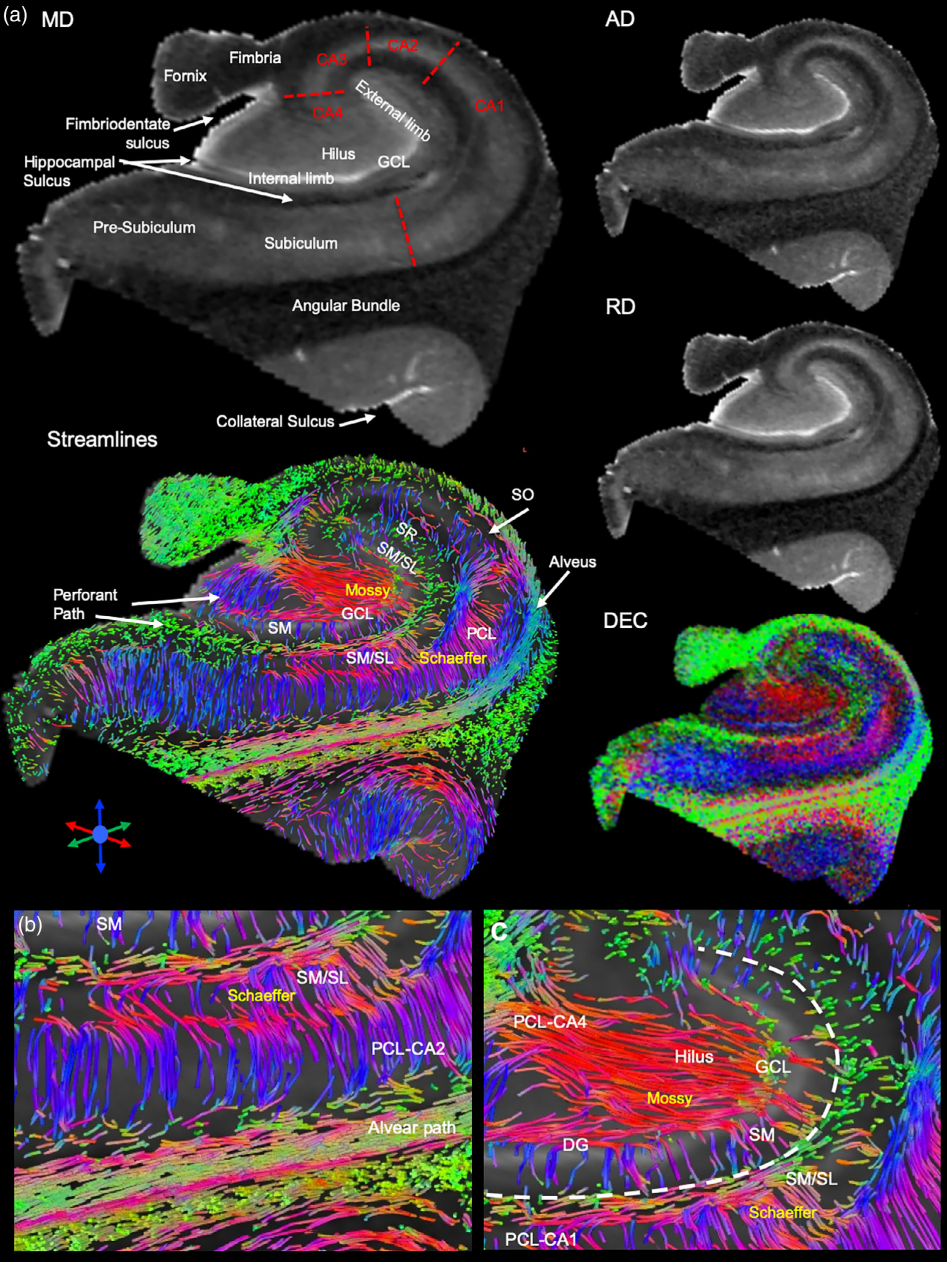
Diffusion MR imaging and regional connectivity. (a) Scalar indices consisting of mean diffusion (MD), axial diffusion (AD) and radial diffusion (RD) define cell layers, such as the granule cell layer (GCL) of the dentate gyrus (DG), as well as the pyramidal cell layer (PCL) that can be sub‐divided in cornu ammonis (CA) 1–4 regions. Tractography of the sample and diffusion encoded color (DEC) images of the fractional anisotropy (FA) further reveal fiber connections between different cell layers. (b) Tractography affords a further dimension of anatomical information that is not discernable from scalar index images that affords the assessment of intra‐hippocampal connectivity. (c) A detailed view of connections, including Mossy fibers and Schaeffer collalerals, afford a system's analysis between different hippocampal layers and regions
